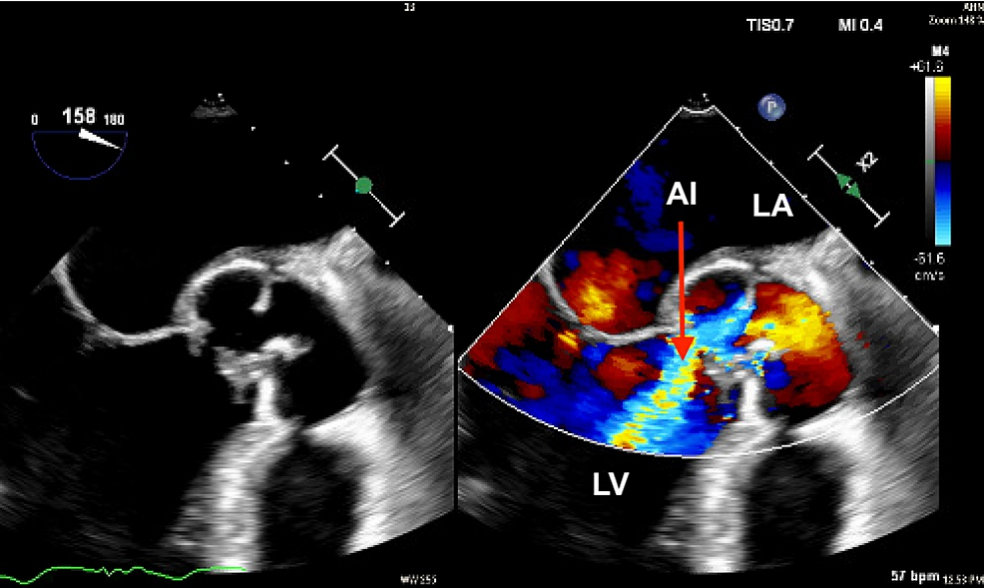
Transesophageal echocardiographic findings of aortic root abscess with severe aortic insufficiency (red arrow). AI: aortic insufficiency; LV: left ventricle; LA: left atrium
